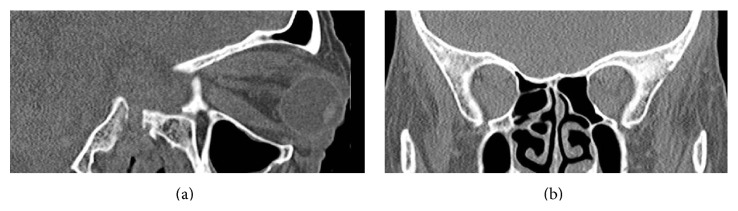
(a) Sagittal CT scan showing severe exophthalmos. (b) Coronal CT scan: apical crowding causing bilateral DON.
